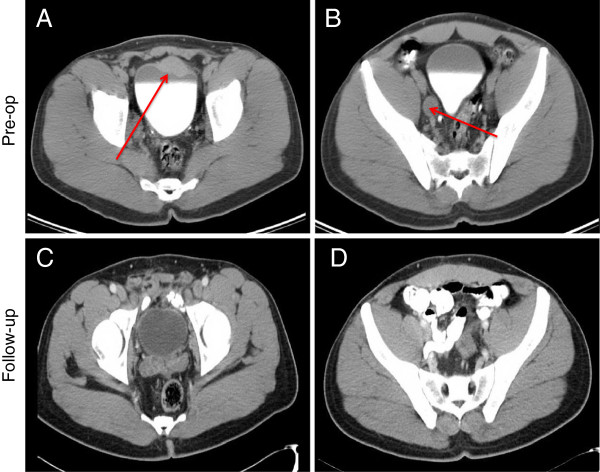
Contrasted enhanced CT axial images of the pelvis. (A) Initial bladder tumor measuring 3.7 cm by 1.8 cm, (B) enlarged right pelvic lymph node (other cuts demonstrated bulky bilateral pelvic adenopathy), (C) post-operative imaging (12 months) no bladder tumor evident and (D) post-operative imaging (12 months) no pathologic pelvic adenopathy.
